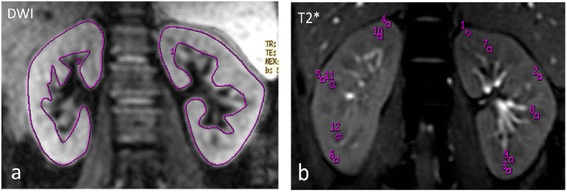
Renal functional MR imaging of Patient A with LN-IV(S)A. (a) DWI imaging. (b) T2* imaging. The proteinuria was 1.3 g/24 h and serum creatinine was 52 μmol/L, the renal pathology AI was 12 and CI was 0. Renal BOLD images showed a clear demarcation between cortex and medulla. The ADC value of left kidney was 2.81 x10^−3^ mm^2^/s and that of right kidney was 2.78 x10^−3^ mm^2^/s, the R2* values were similar to normal kidneys.
